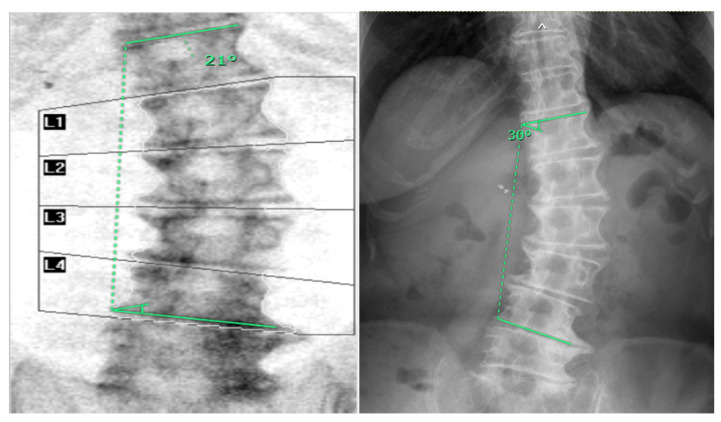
Measurement of the Cobb angle from lumbar DXA images and spine radiographs.
